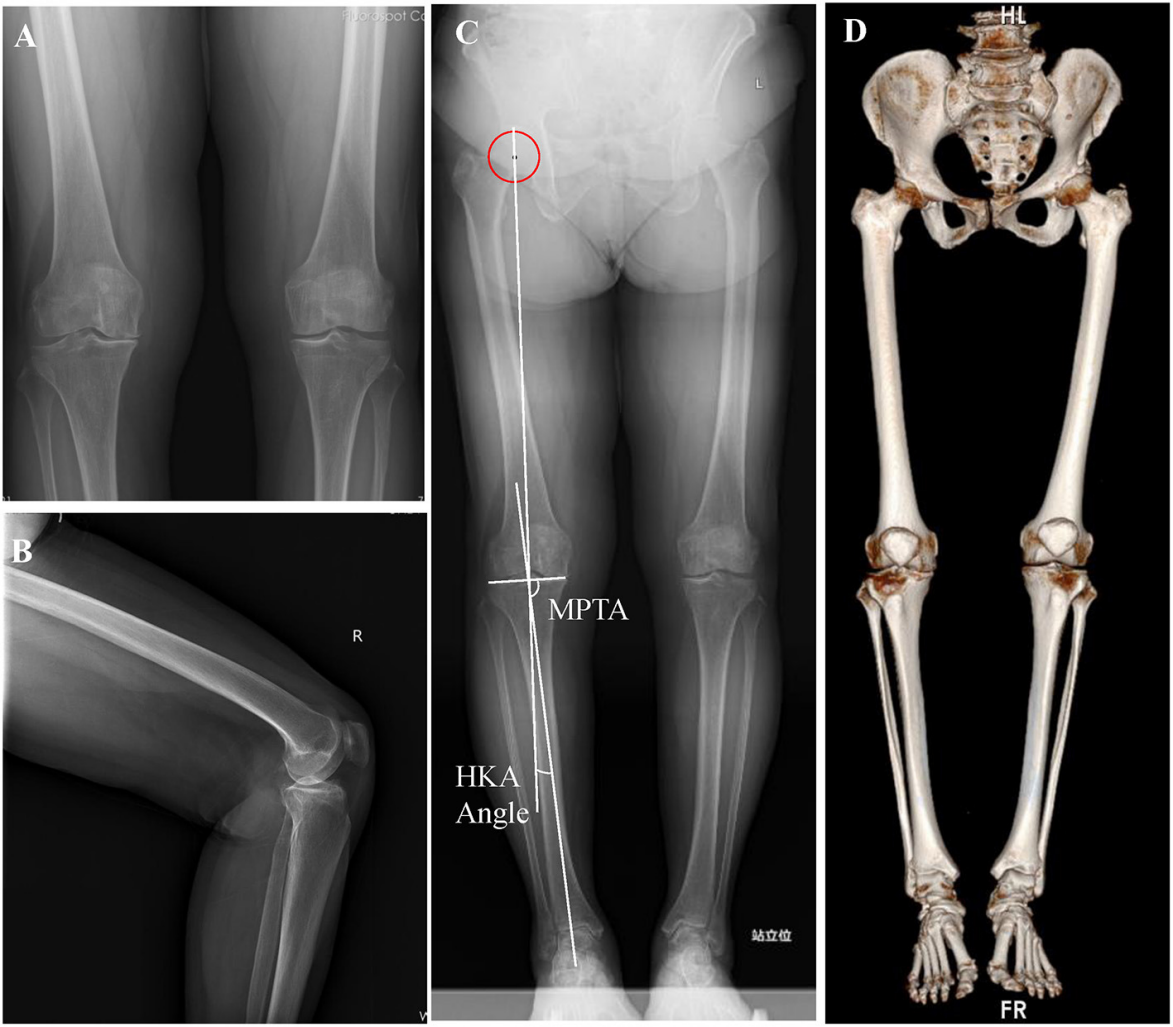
Pre-operative imaging data. (A) Anterior film of bilateral knee joints. (B) Lateral film of the right knee joint. (C) Full-length film of both lower limbs in heavy position. (D) CT 3D reconstruction of both lower extremities and pelvis.
